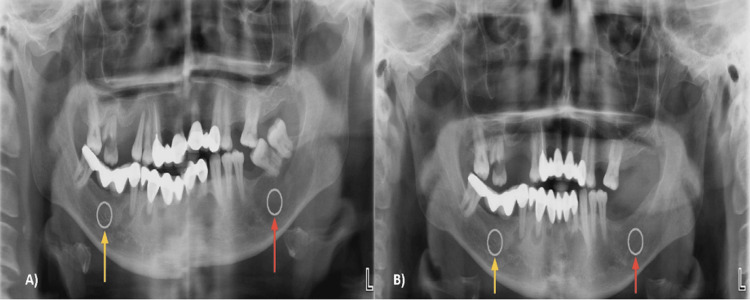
Preoperative (A) and postoperative (B) panoramic radiographs demonstrating standardized ROI placement. Yellow arrows indicate the contralateral control region, while red arrows indicate the lesion area used for grayscale analysis. ROI, region of interest
